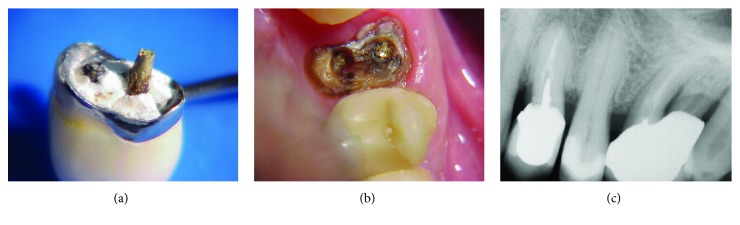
(a) Initial aspect of the metal-ceramic crown with a fractured cast core; (b) aspect of the fractured dental remnant of the cast core inside the root canal, which was later removed; (c) initial radiography.
